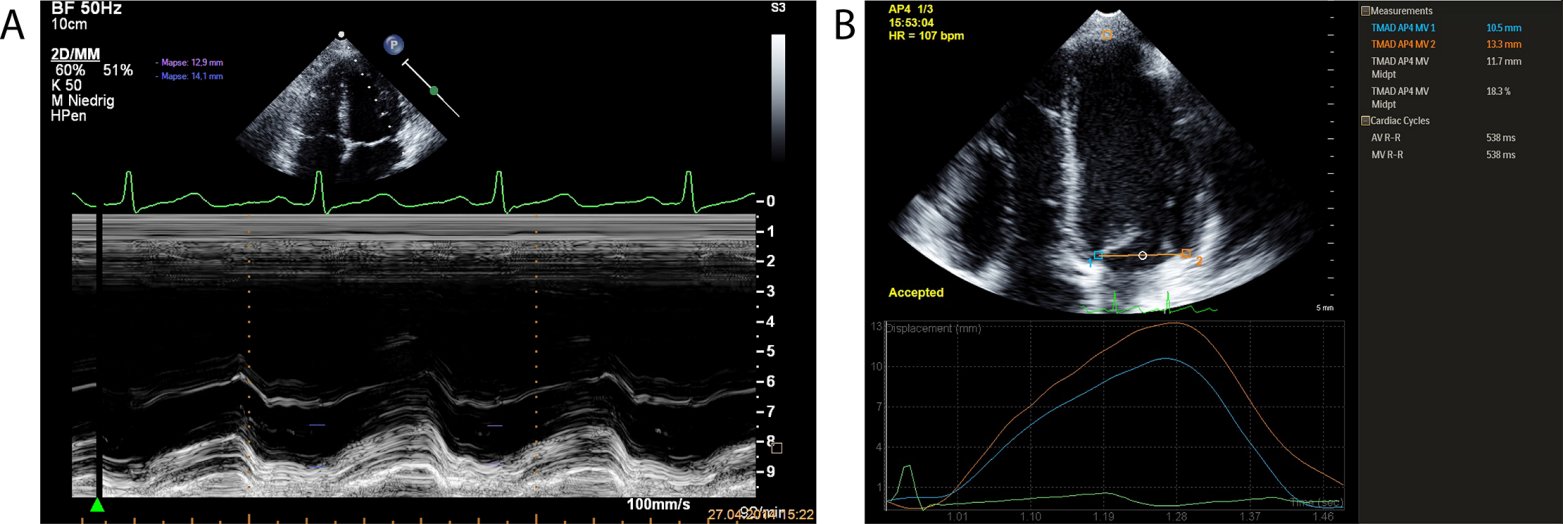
Echocardiographic image samples of MAPSE imaging. A. M-mode derived MAPSE measurement, B. B-mode derived MAPSE measurement.
